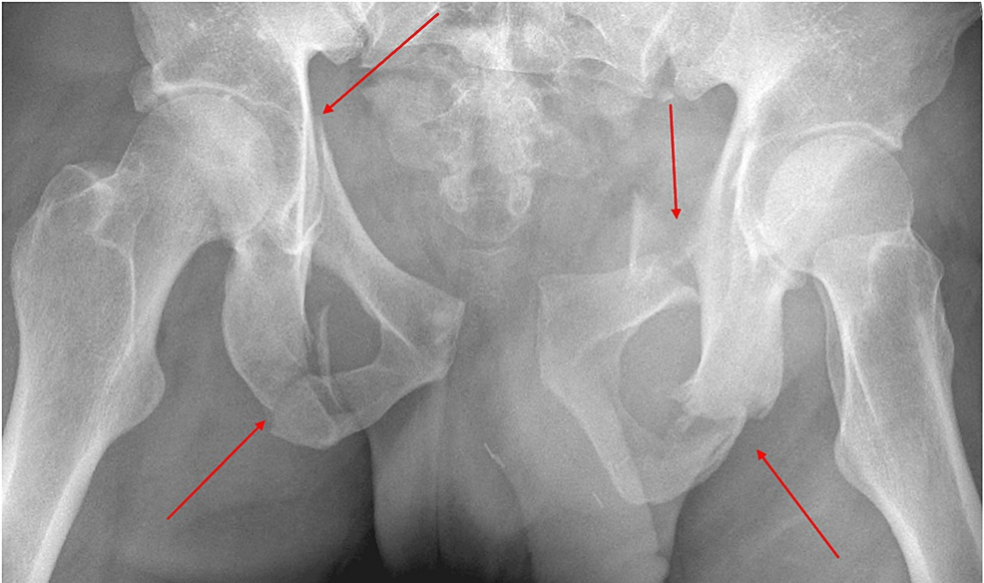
Pelvis radiograph. A single frontal radiograph of the pelvis was obtained. Markedly displaced fractures were noted through the bilateral superior and inferior pubic rami with gross distraction of the pubic rami sacroiliac joint. Distraction was also suspected, with fractures extending through the S3 vertebral body. Points of fracture are indicated with red arrows.
